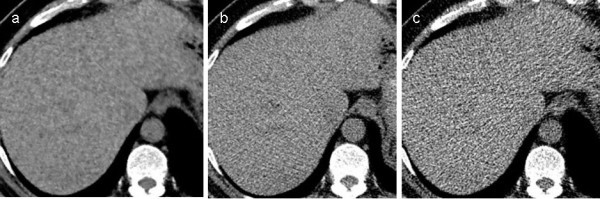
Blotchy pixelated appearance of each CT image. Axial unenhanced CT images of UL-MBIR (a), L-ASIR (b), and UL-ASIR (c) of a 61-year-old man weighing 75 kg, the same patient as in Figure 1. A blotchy pixelated appearance is uniquely seen in UL-MBIR images.
